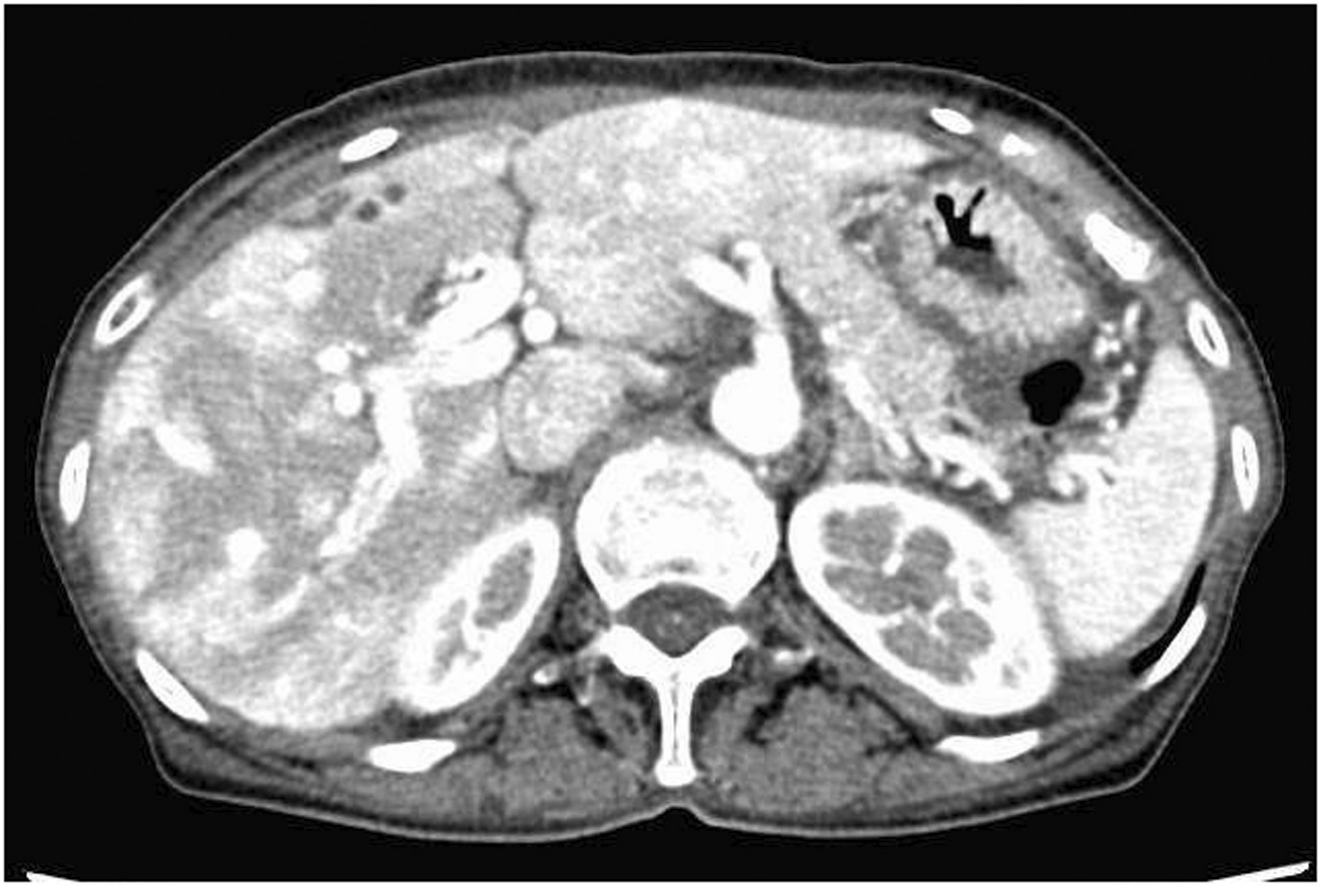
Contrast‐enhanced CT showed multiple hepatic arteriovenous malformations.
